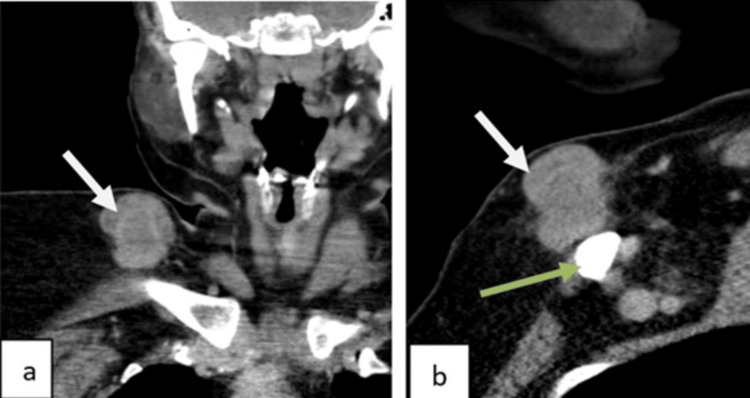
Coronal (a) and sagittal (b) venous phase CT sections show a well-defined heterogeneously enhancing right supraclavicular lesion with areas of necrosis within. No invasion of the right clavicle (green arrow) is noted. Fat planes are maintained with the surrounding structure. CT: computed tomography
